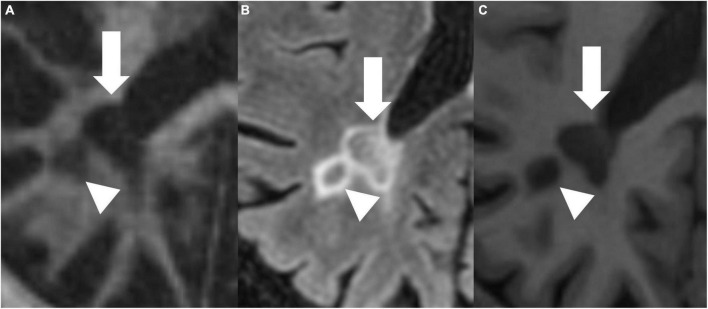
IR-UTE (A), FLAIR (B), and MPRAGE (C) images show two periventricular MS lesions (white arrow and white arrowhead) next to each other.
